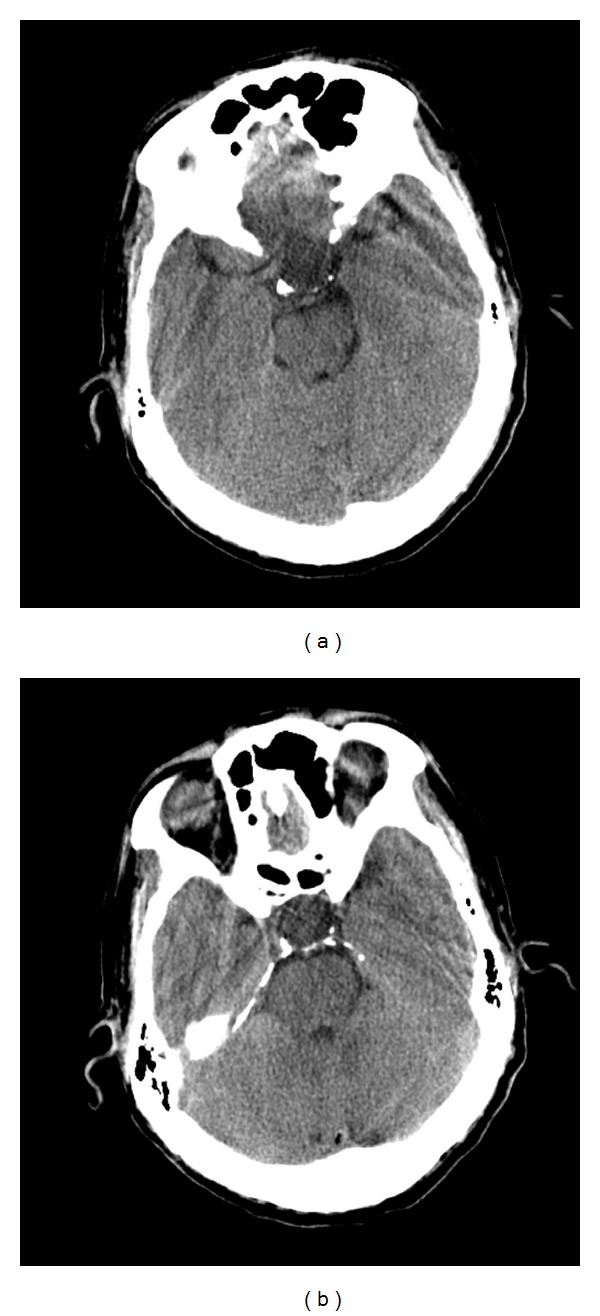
Noncontrast head CT in May revealing an enlarged sella turcica without evidence of calcifications, most consistent with a sella-suprasellar cystic mass.
